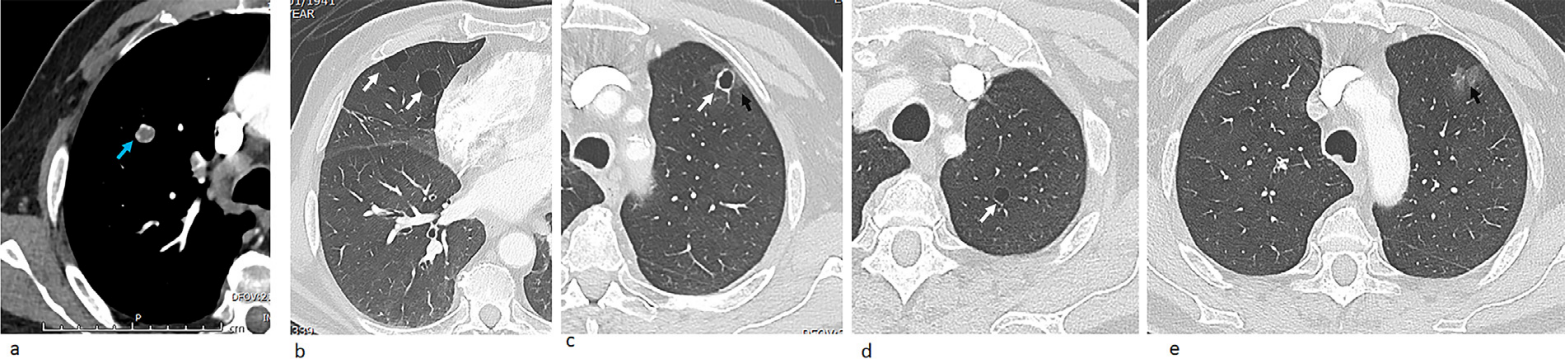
An 80-year-old male with a metastatic angiosarcoma of the scalp. Contrast enhanced axial (A-E) sections of lung CT scan showed multiple thin-walled cysts (white arrows) accompanied with ground-glass attenuation which are likely marking areas of hemorrhage (black arrows). A solid pulmonary nodule with stippled calcifications and enhancing rims is noted in the right upper lung lobe (blue arrow). These lesions represent pulmonary metastatic deposits of the scalp angiosarcoma (Color version of the figure is available online.).
